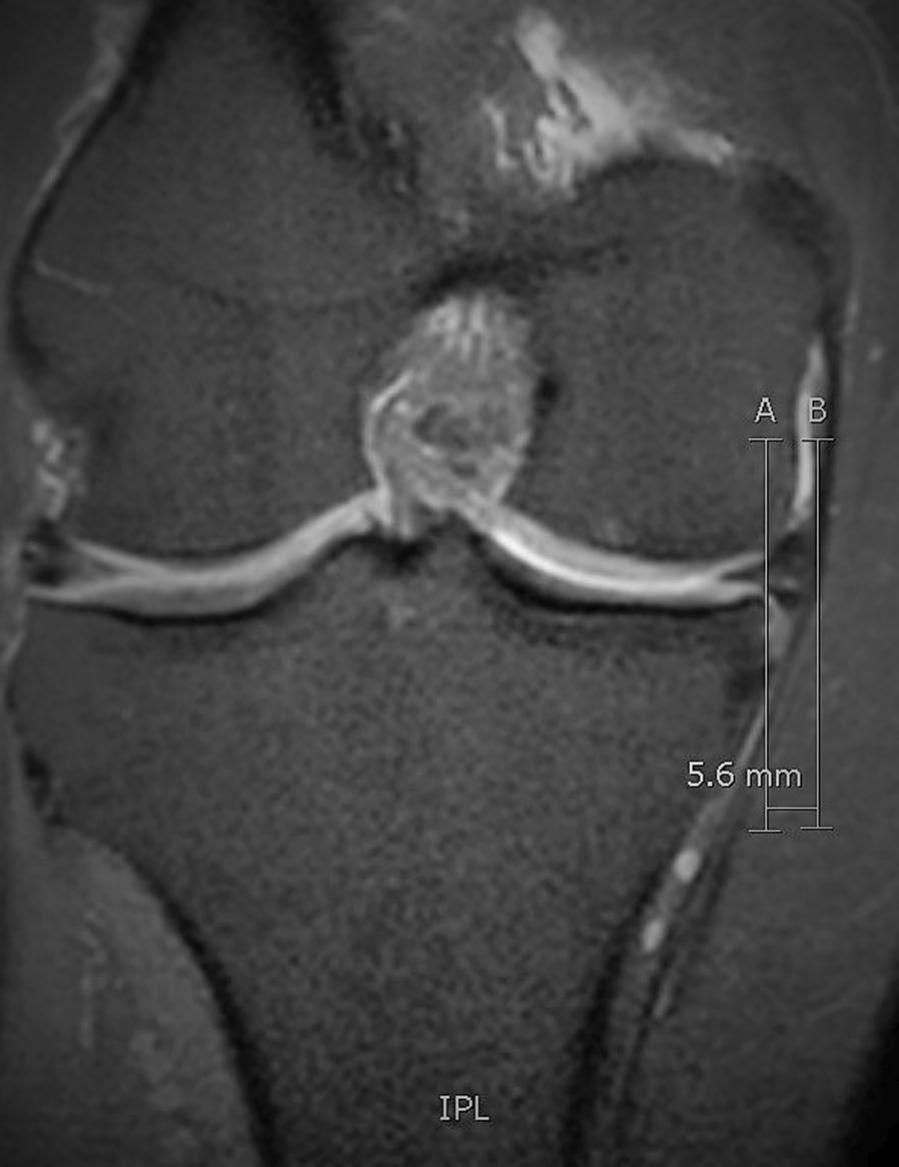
Magnetic resonance imaging (MRI) mid-coronal view of the right knee, where the tibial eminences are most prominent. Medial meniscal extrusion (MME) is measured 5.6 mm
